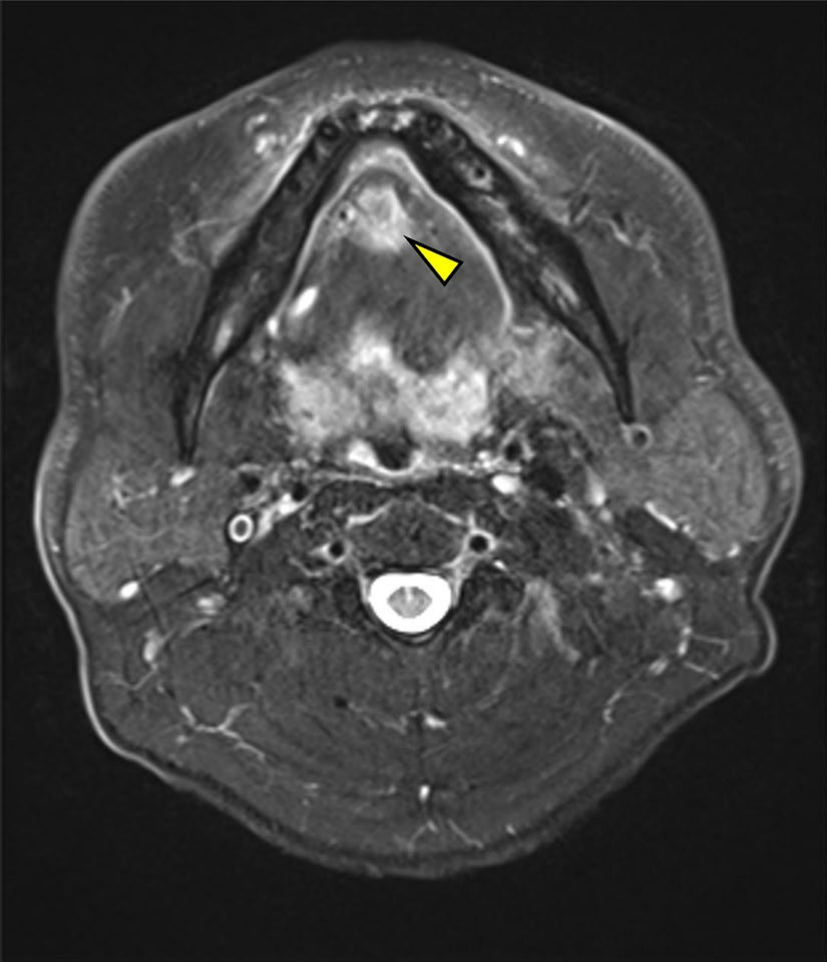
T2-weighted magnetic resonance (MR) image showing median lingual lymph node metastasis (LLNM) (arrowhead) in the lingual septum.
